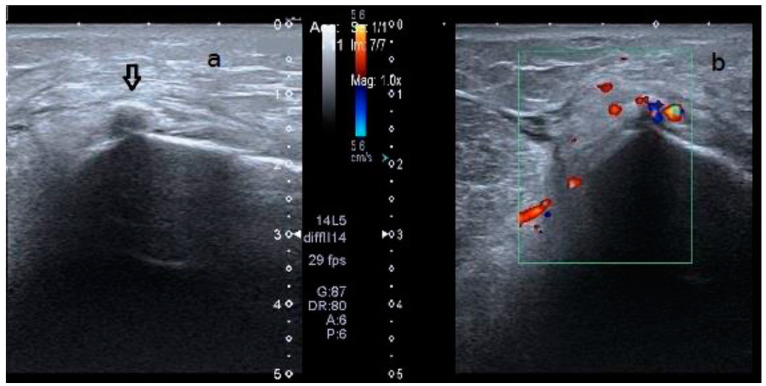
(a) Long-axis sonogram showing an echogenic focus with posterior acoustic shadow overlying or adjacent to the internal femoral condyle (black arrow). Black Arrow = needle. (b) Hypervascularization is observed in the color Doppler scan.
